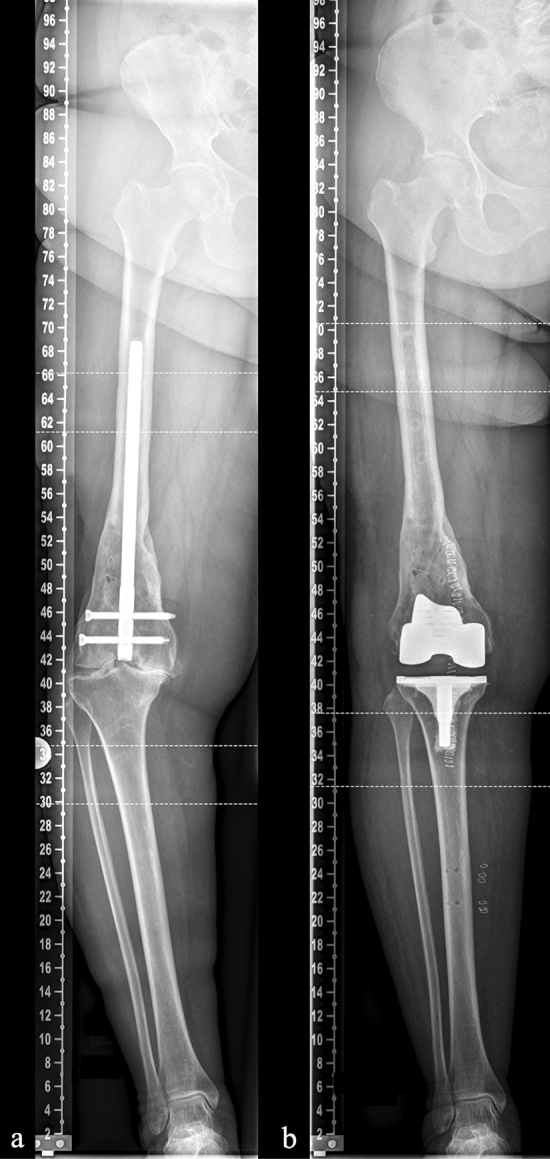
Complex rTKA in a case of post-traumatic osteoarthritis with retained hardware requiring removal during the TKA procedure. a Preoperative anterior − posterior weight bearing long leg view in a case of femoral fracture treated with retrograde intramedullary nailing. b Postoperative radiographs after rTKA
